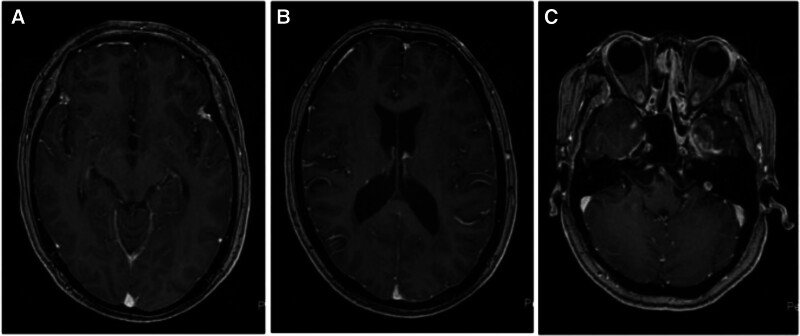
MRI of the patient’s brain at the time of confirmed leptomeningeal metastases: (A, B) meningeal enhancement, (C) left temporal lobe, and peripheral edema. MRI = magnetic resonance imaging.
